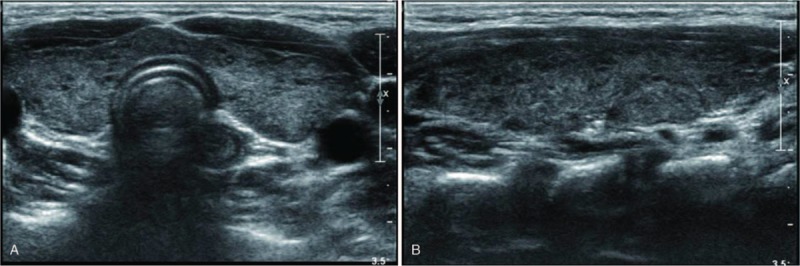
(A) Transverse and (B) longitudinal ultrasonographic images of Grade 2.
